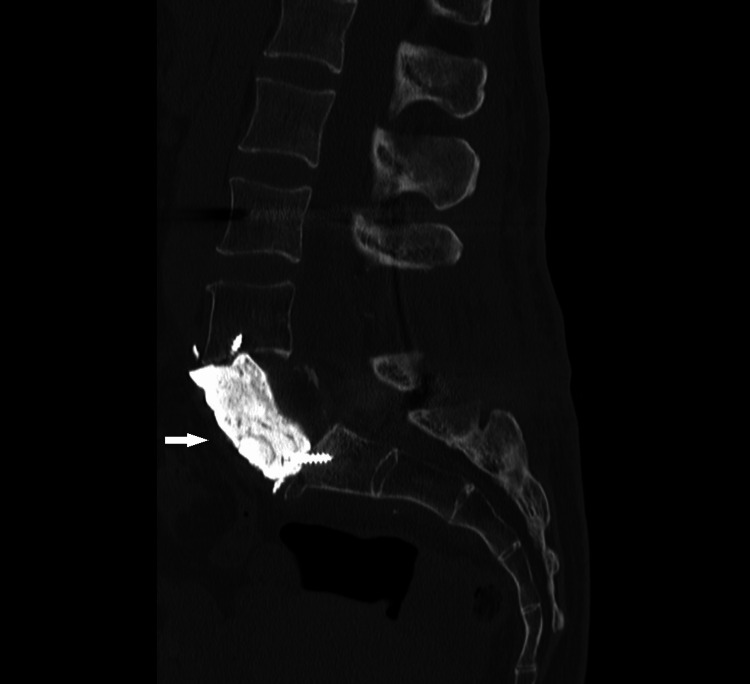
Lumbar spine CT scan after ventral corpectomy with reconstruction of the defect with cementoplasty.
